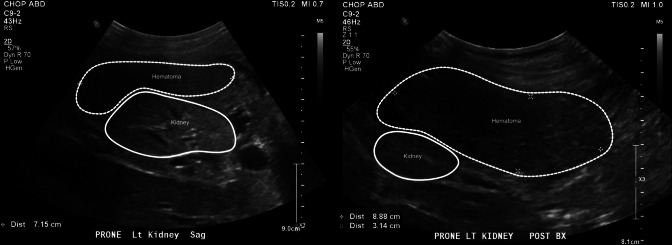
Post-renal biopsy renal ultrasound images of the kidney and hematoma
